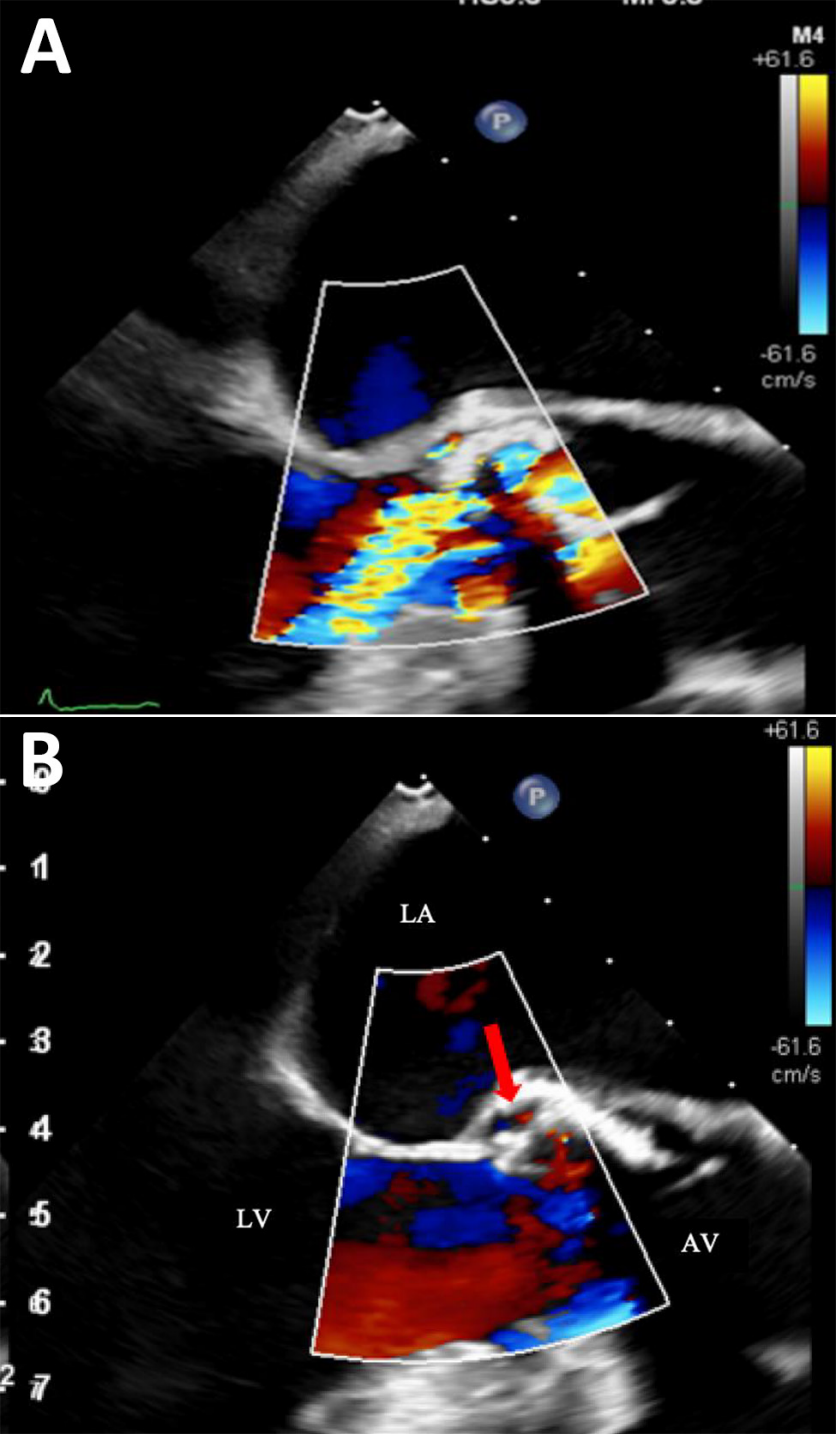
Color doppler echocardiography images from fatal case of subacute native aortic endocarditis, Geneva, Switzerland. A) Mid-esophageal long-axis view during diastole, showing moderate to severe aortic regurgitation. B) Mid-esophageal long-axis view with left ventricular chamber, aortic valve, and aortic root during systole. Red arrow indicates systolic flow with the pseudo-aneurysm. AV, open aortic valve; LA, left atrial; LV, left ventricle.
